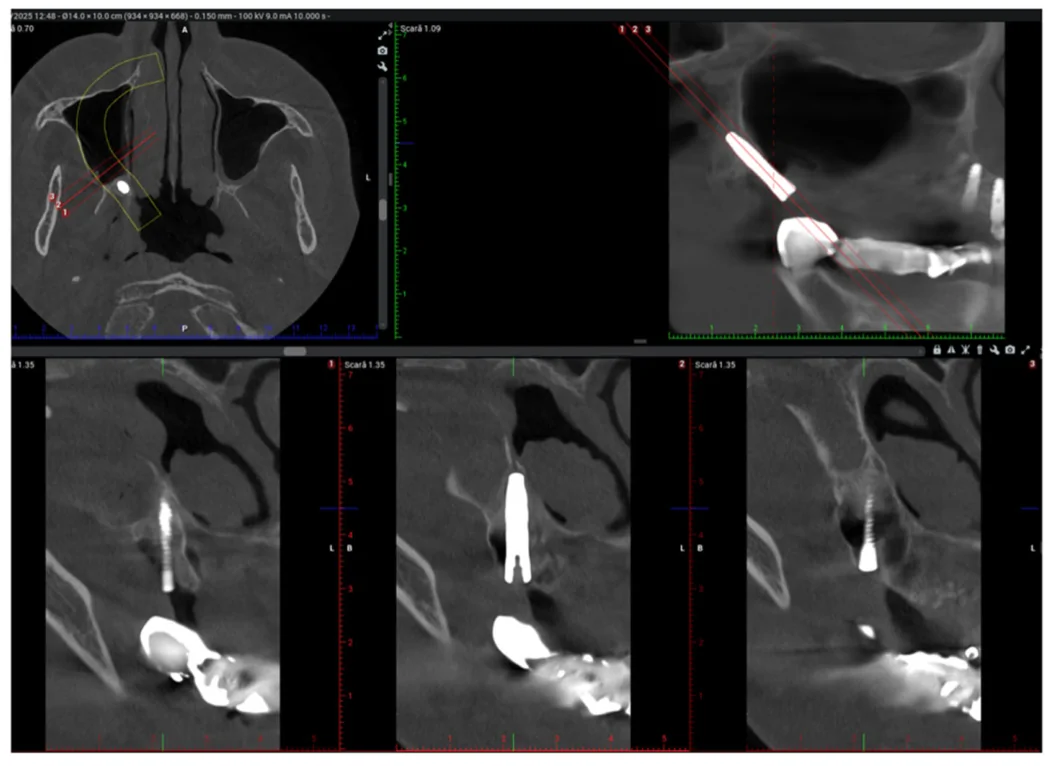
Immediate postoperative CBCT confirming proper positioning of the newly placed right pterygoid implant.
